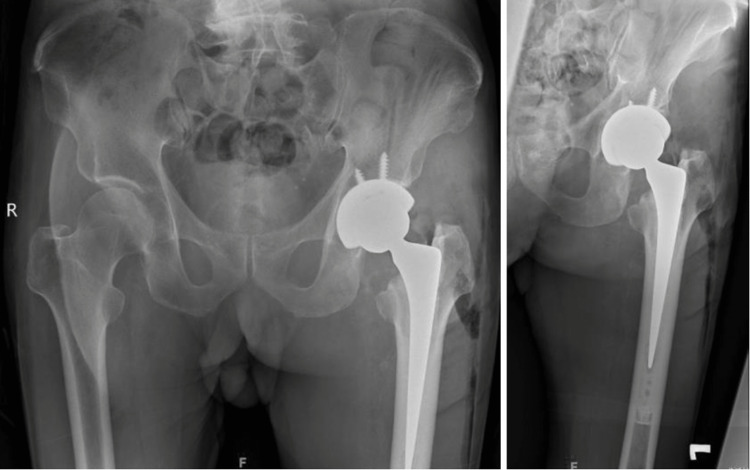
Post-operative AP pelvis and AP left hip radiographs showing a satisfactory left total hip replacement using the modified SPAIRE approach.
